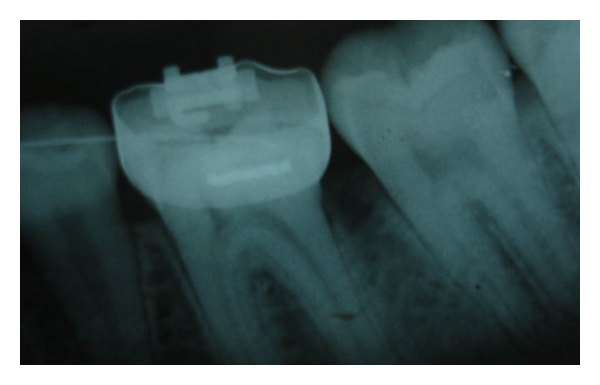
Radiographic view one month following treatment demonstrating damage to the alveolar bone between the first and second lower left molars.
